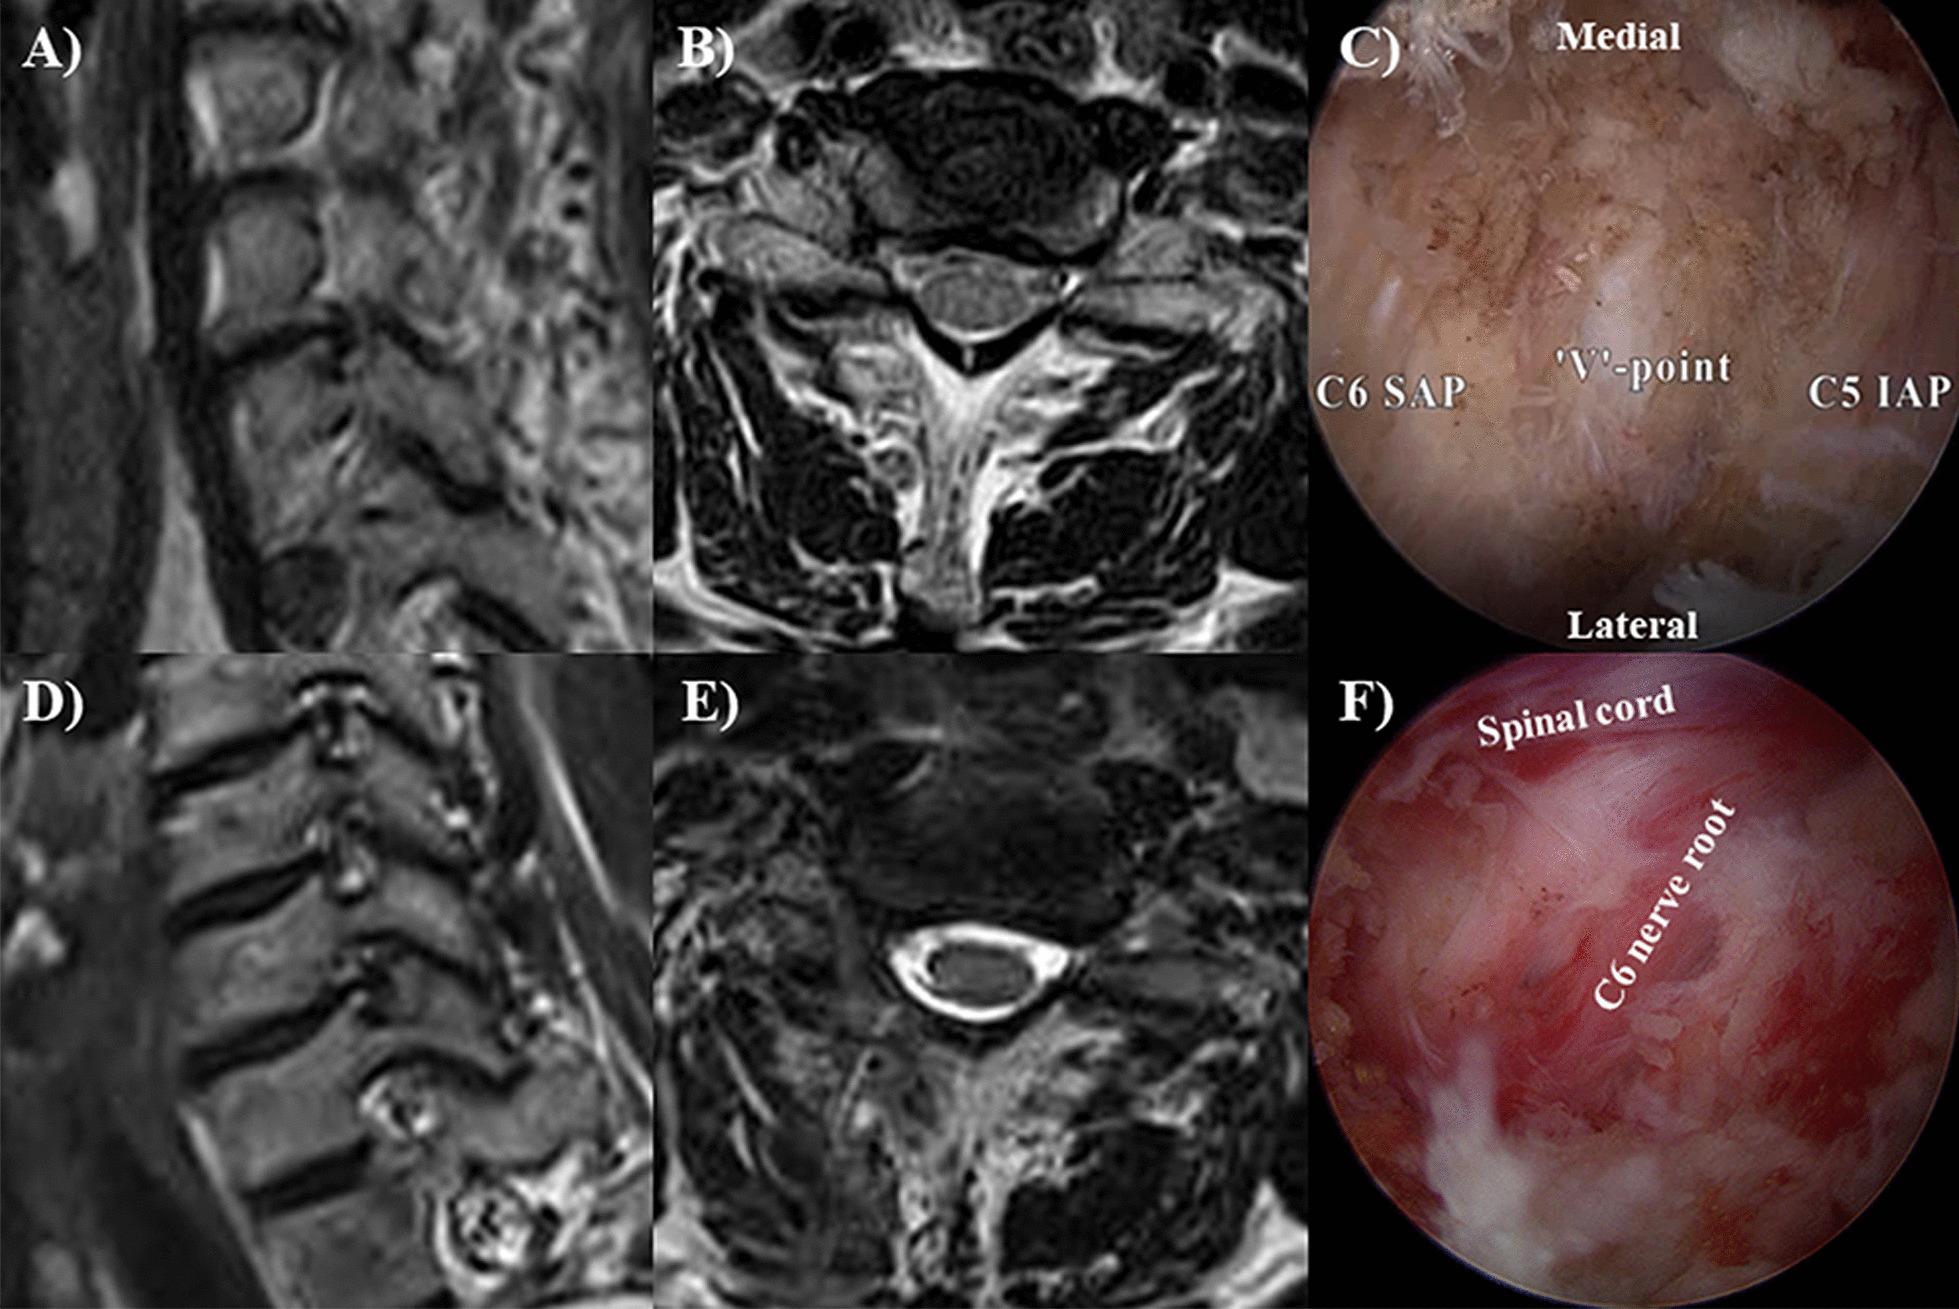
Biportal full-endoscopic posterior cervical foraminotomy that occurred in a 64-year-old woman. The foraminotomy occurred 2 months previously in the 64-year-old woman who had radiating pain and motor weakness in the right upper extremity. T2-weighted A right oblique and B axial images of pre-operative magnetic resonance imaging (MRI) demonstrating right C5-6 foraminal stenosis; C Intraoperative endoscopic image of the “V”-point; F After circumferential foraminotomy is performed from the “V”-point, fully decompressed thecal sac and C6 nerve root are found; D, E Post-operative MRI showing well-decompressed nerve root with minimally damaged paraspinal muscles. The patient’s symptoms also improved
